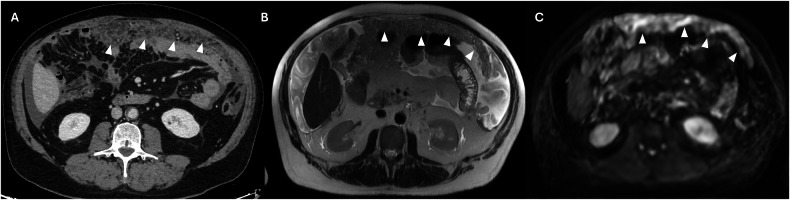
Ovarian cancer with peritoneal carcinomatosis in a 72-year-old woman. CT portal-venous phase (A), MRI T2w and DWI (b = 800 mm^2^/s) (arrowheads) (B, C), axial plane showed diffuse involvement of omental fat known as “Omental cake” pattern (arrowheads)Mass-like pattern: this pattern involves soft-tissue masses of several centimeters, resulting from the confluence of multiple nodular implants. Masses more than 10 cm in diameter are called “bulky tumors.”Teca pattern: This involves significant engagement of the visceral peritoneum lining the loops of the small bowel, causing thickening, distortion, and fixation of the mesentery. This culminates in intestinal obstruction, a “frozen pelvis” (Fig. 8) [30].
